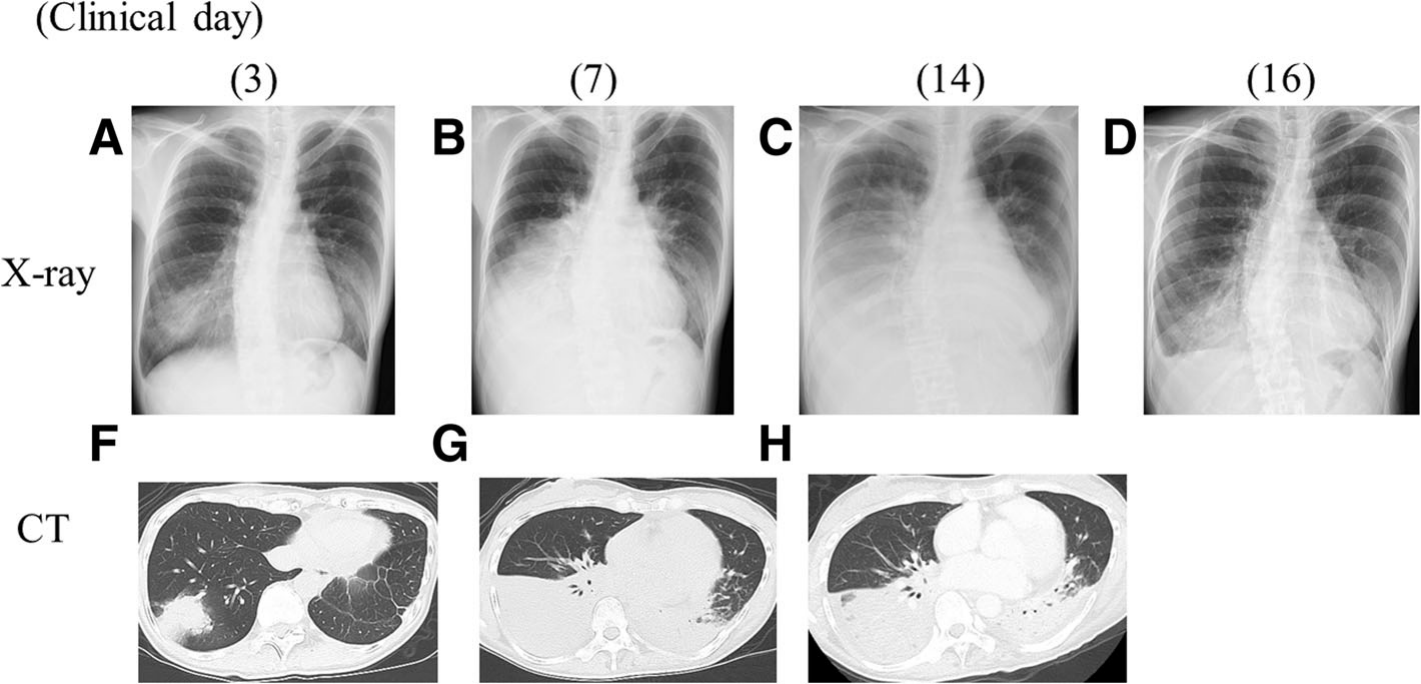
Chest X-ray image (A, B, C and D) and chest CT image (F, G and H) of the thorax. 10 days before admission to our hospital (clinical day 3), the chest images revealed sub-segmental infiltration in the right lower lobe, which enlarged to bilateral lower lung field from clinical day 7 to 14. After switching the antibiotics, the chest images improved until clinical day 16
